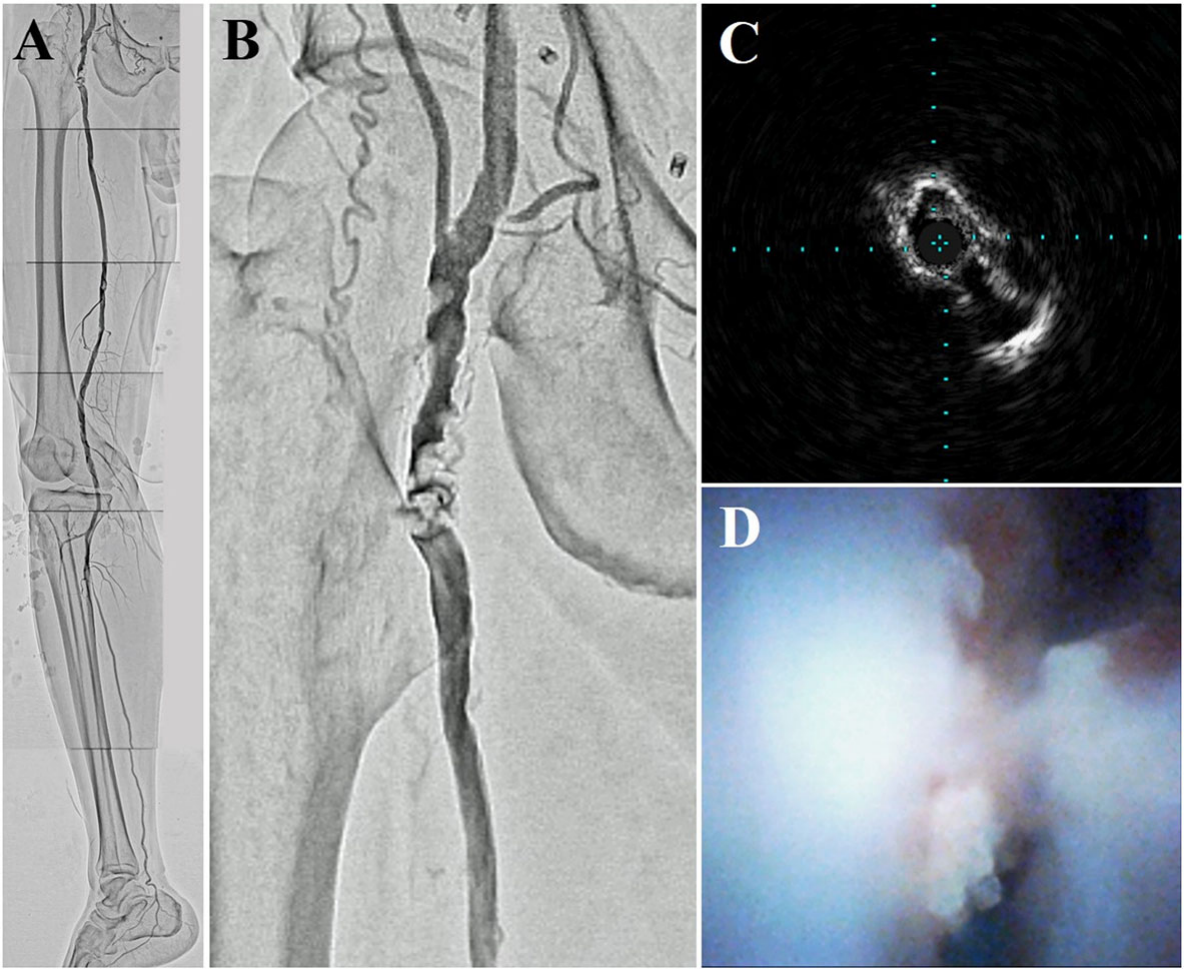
A,B Initial angiography showing a filling defect of contrast medium in the common femoral artery and one straight line through the posterior tibial artery. Initial intravascular ultrasonography (C) and angioscopy (D) images demonstrating a calcified mass protruding into the vascular lumen
